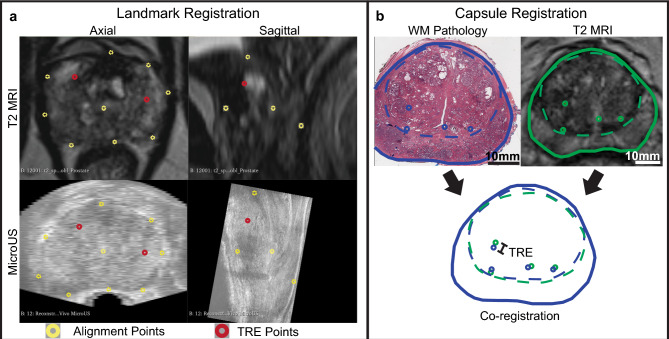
(a) Landmark registration between microUS and MRI. Points along the urethra and the capsule were selected to inform the TPS transformation (yellow). The distance between five internal anatomical fiducials (red) were used to evaluate TRE. (b) Capsule registration between MRI and WM pathology. The distance between fiducials (circles) were used to evaluate TRE and the overlap of the transition zone (dashed lines) was used to determine DICE scores.
